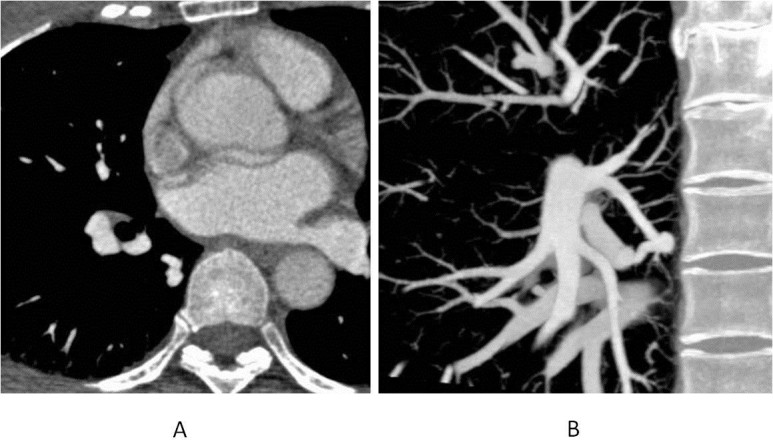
Pulmonary arteriovenous fistula (PAVF) found on the conventional contrast-enahnanced CT. A: On axial image of contrast-enhanced CT, aneurysmal sac of PAVF was noted at S6 of right lower lobe. B: Para sagital oblique view of partial maximum intensity projection (MIP) shows the anomalous continuation of pulmonary artery and vein with aneurysmal sac.
